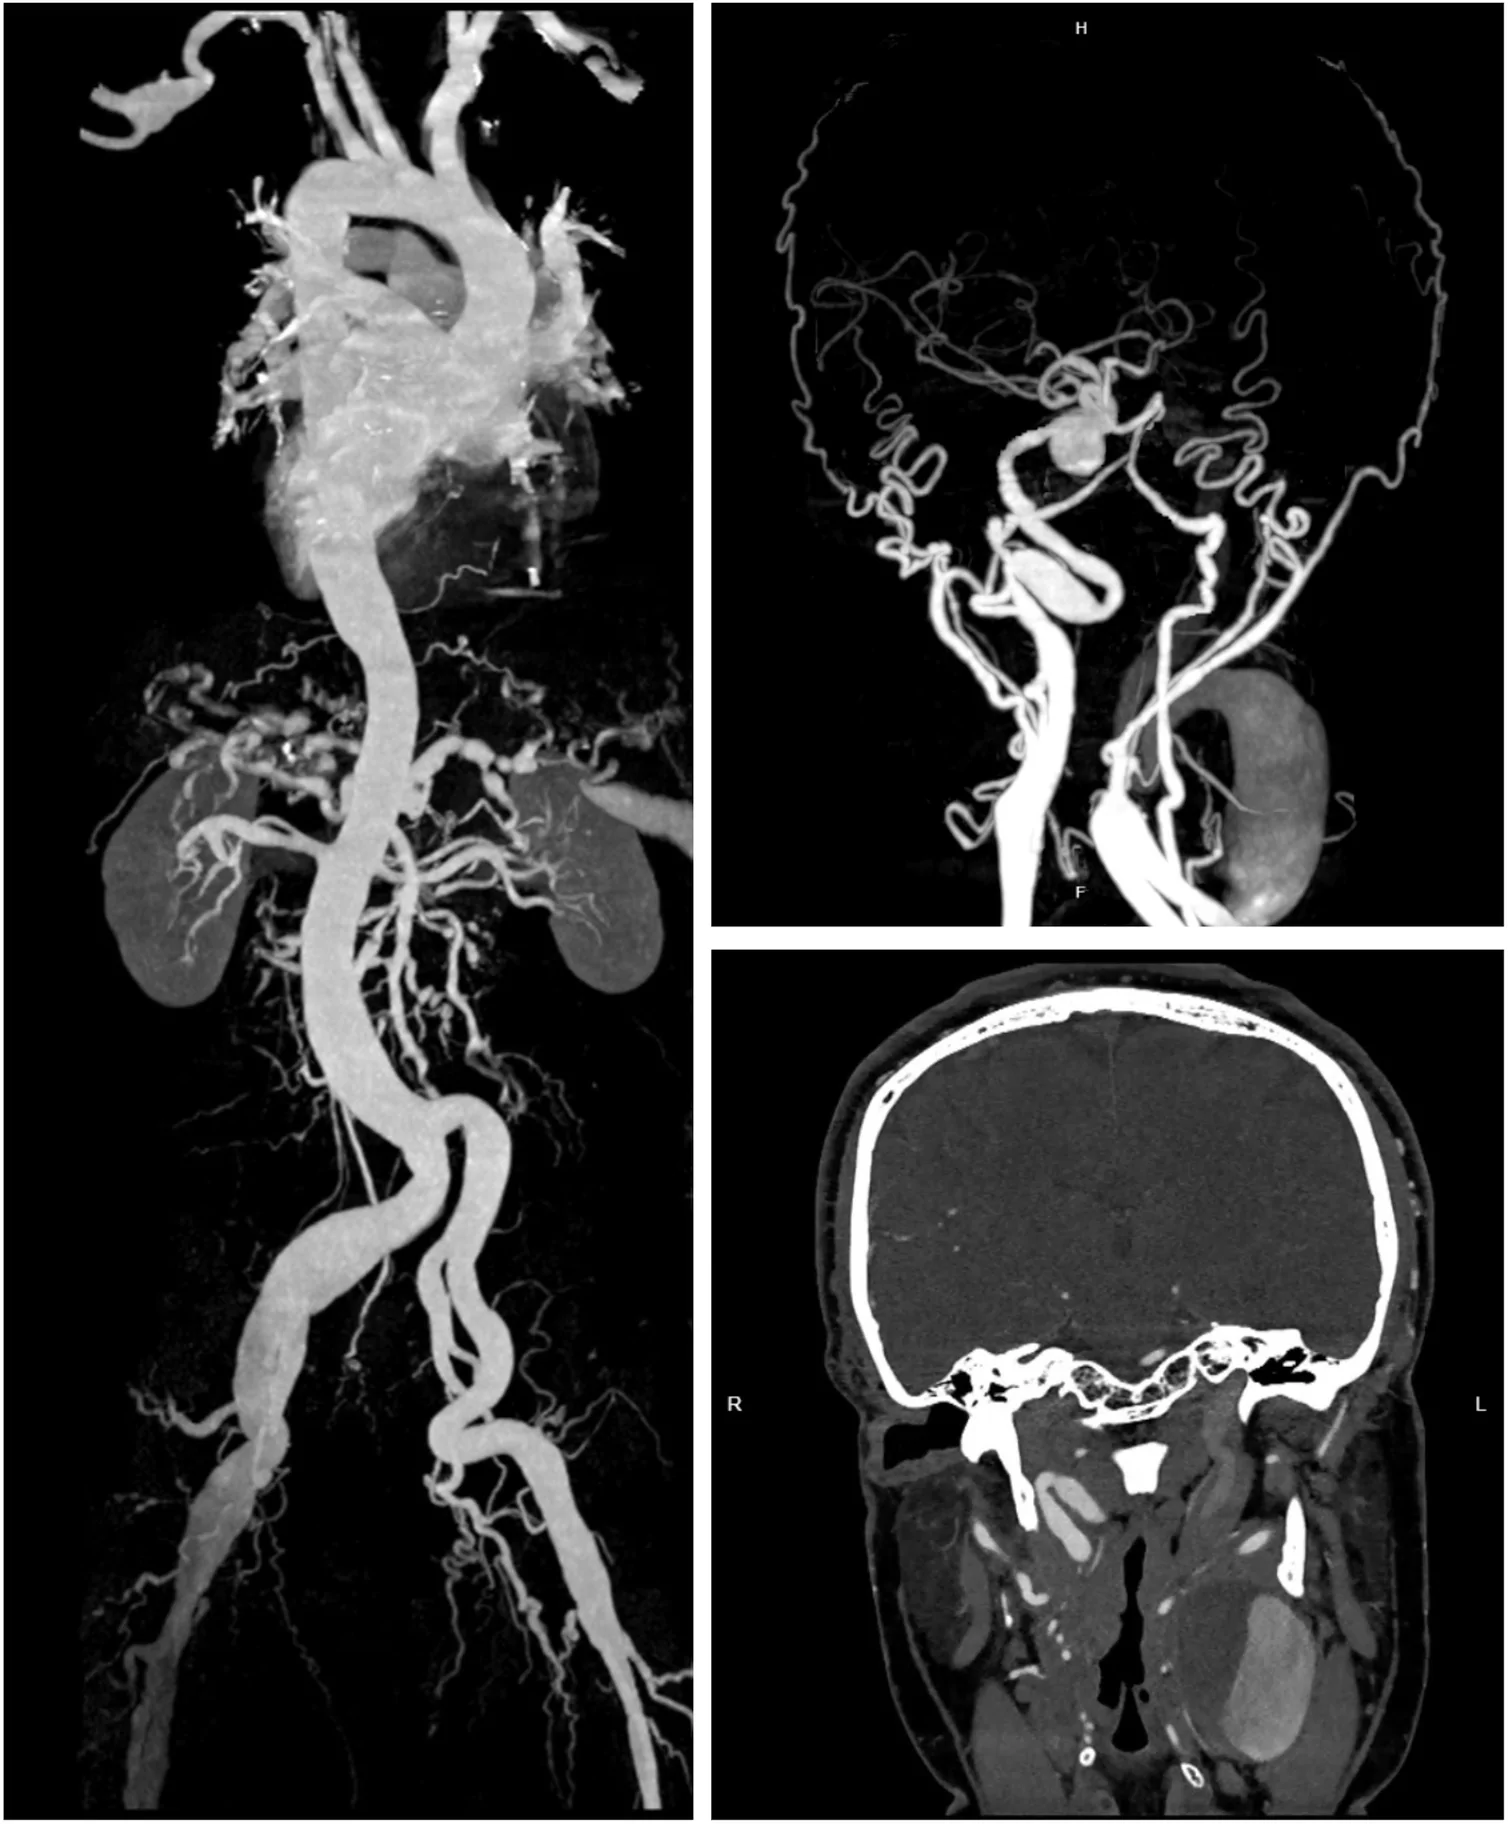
Right: 3D brain CT angiography reconstruction. Giant partially thrombosed aneurysm arising from the cervical segment of the left ICA, located near the carotid bifurcation, associated with marked flow reduction to the intracranial ICA. Additional findings included a large cavernous-segment aneurysm of the right ICA. Left: 3D Abdominal CT angiography reconstruction. Incidental thoracic aortic dissection with features of a DeBakey IIIa/Stanford B. Multiple fusiform aneurysms in the arteries of the celiac trunk and superior mesenteric artery, both common iliac arteries, and left femoral artery, plus aortoiliac dolichoectasia.
